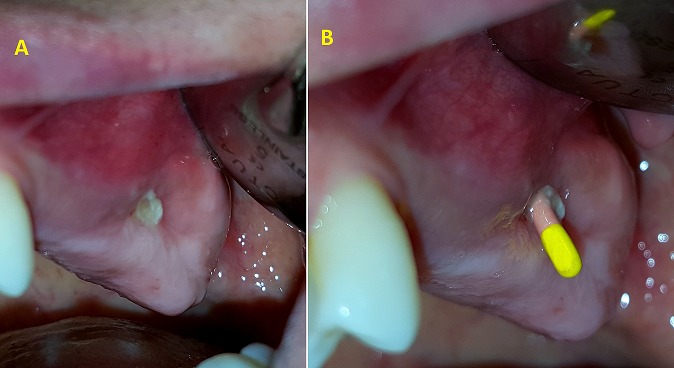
A) intraoperative picture showing oroantral fistula; B) use of the gutta-percha cone to explore the fistula by X-ray
